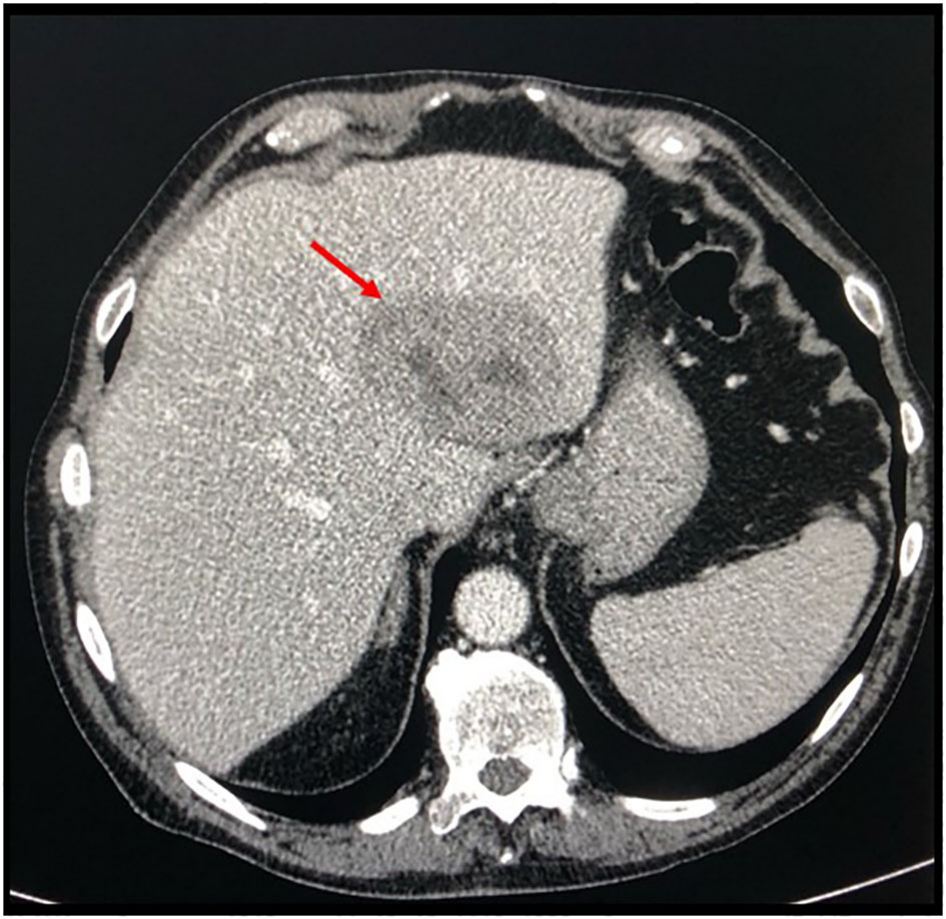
Basal axial CT scan evaluation, showing a hypodense and partially colliquated liver lesion at segments II–III (*arrow*).
